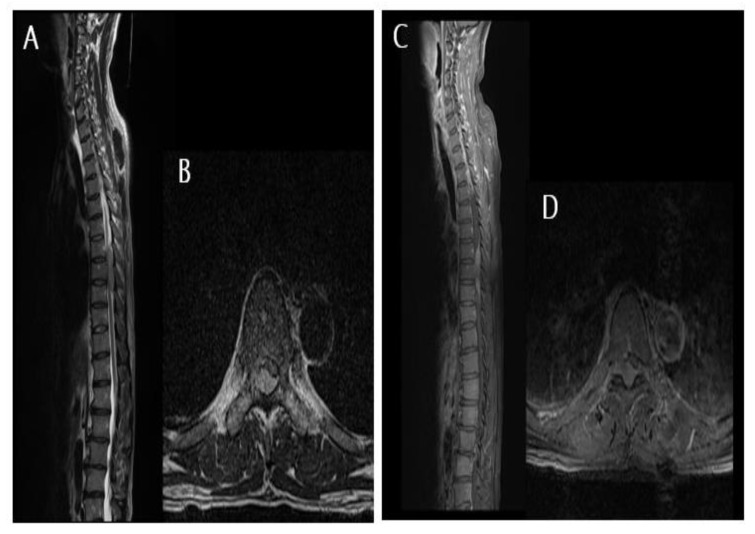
Magnetic resonance images of the patient. (A,B) T2-weighted sagittal and axial images showing an epidural mass lesion compressing the spinal cord from T7 to T9. (C,D) Contrast-enhanced magnetic resonance images reveal homogeneous enhancing epidural lesions at T7–T9 levels.
